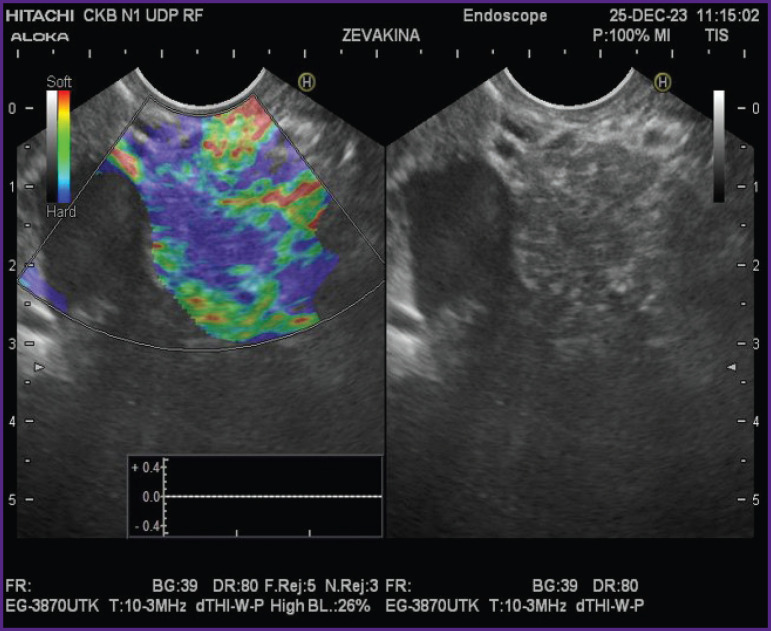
Endoscopic ultrasound examination, elastometry of the pancreas: wide areas of parenchymal fibrosis are seen
